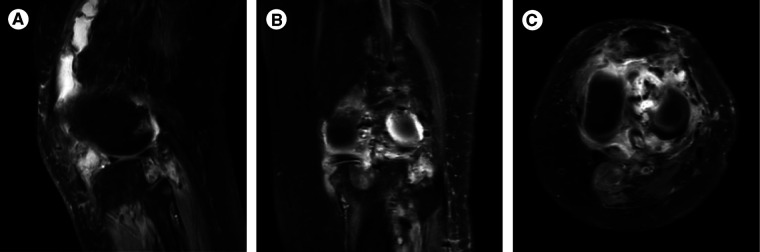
MRI of the left knee joint: multiple abnormal nodules in the articular cavity, posterior tibial space, and popliteal space, which are presented as isosignals on T1WI and mixed signals on T2WI. (A) Median sagittal section. (B) Coronal section. (C) Transverse section.
